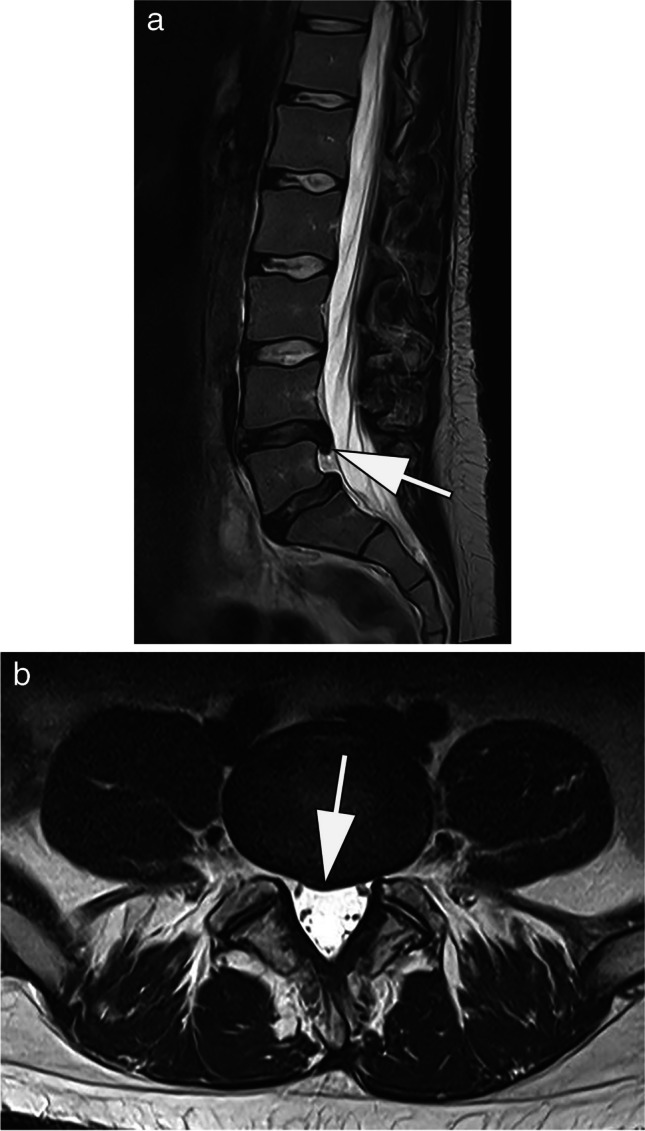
MRI at 0.55 T of the lumbar spine of a 32-year-old patient with non-radiating lower back pain. A T2-weighted sagittal image shows a subligamental disc herniation L4/5 inferiorly protruded (arrow). B Corresponding axial T2-weighted image at the level of the intervertebral space shows median disc protrusion without recessal obstruction (arrow). Acquisition time (TA): 02:48 min (A), 03:35 min (B)
